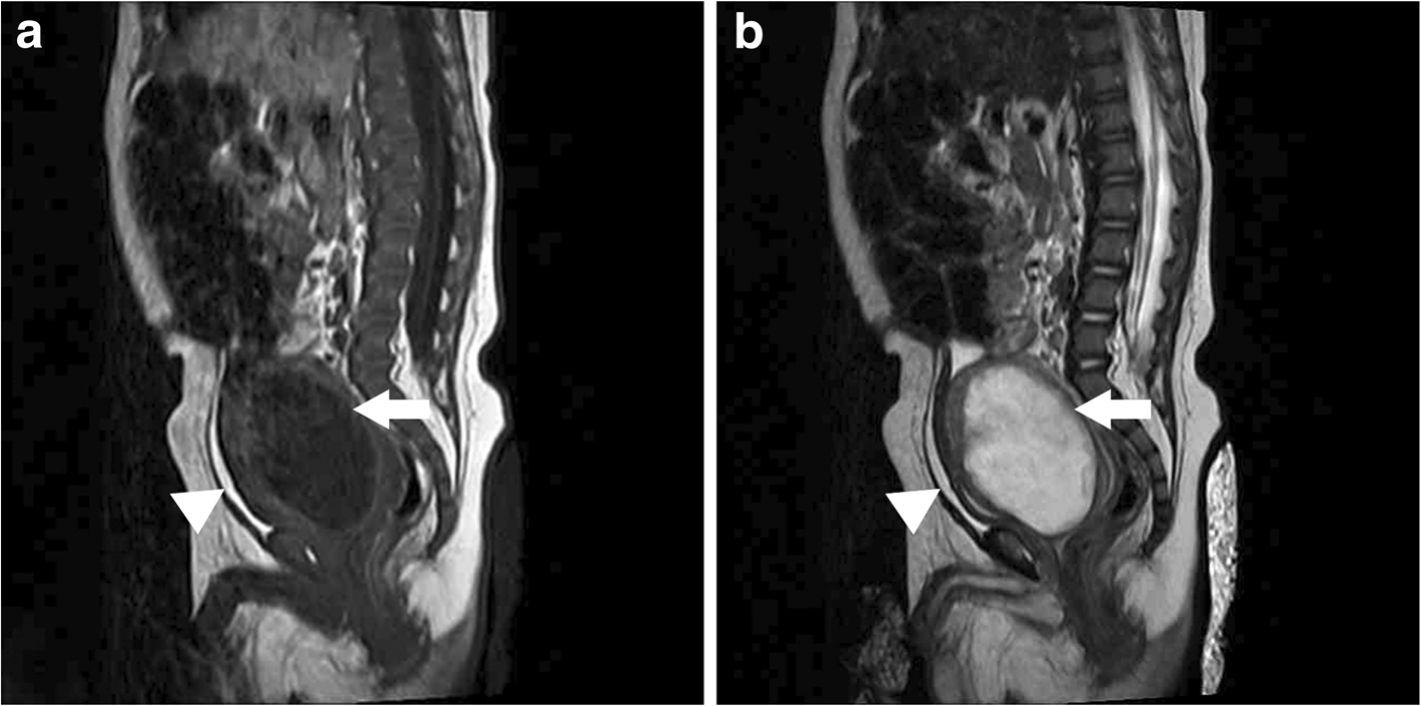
Magnetic resonance image shows a large prostatic utricle (white arrow) behind the urinary bladder (white arrowhead). a Sagittal T1 weighted MR image showing a hypointense lesion (white arrow) with thick and irregular wall posterior to urinary bladder (white arrowhead) and anterior to the rectum. b Sagittal T2 weighted MR image demonstrating a thick-wall, large utricular lesion behind the urinary bladder
